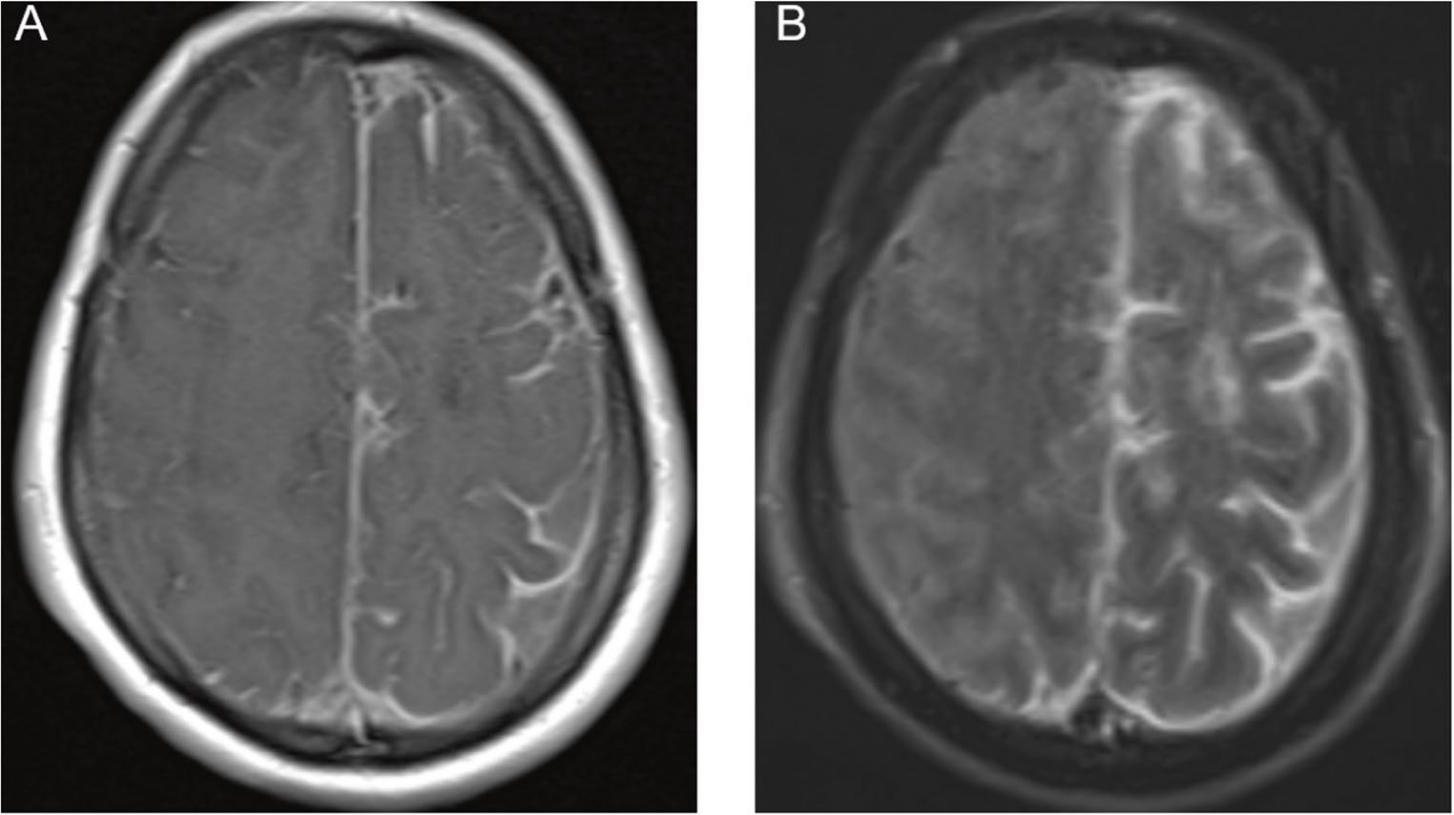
(A) Axial post‑contrast T1‑weighted MRI showing left‑predominant fronto‑parietal leptomeningeal enhancement following a gyral‑sulcal pattern and extending into superficial cortical vessels. (B) Axial post‑contrast FLAIR demonstrating the same topography with greater conspicuity and cortical/subcortical hyperintensity in the right superior and middle frontal gyri, consistent with vasogenic edema adjacent to the involved leptomeninges. Abbreviations: MRI = magnetic resonance imaging; FLAIR = fluid‑attenuated inversion recovery
